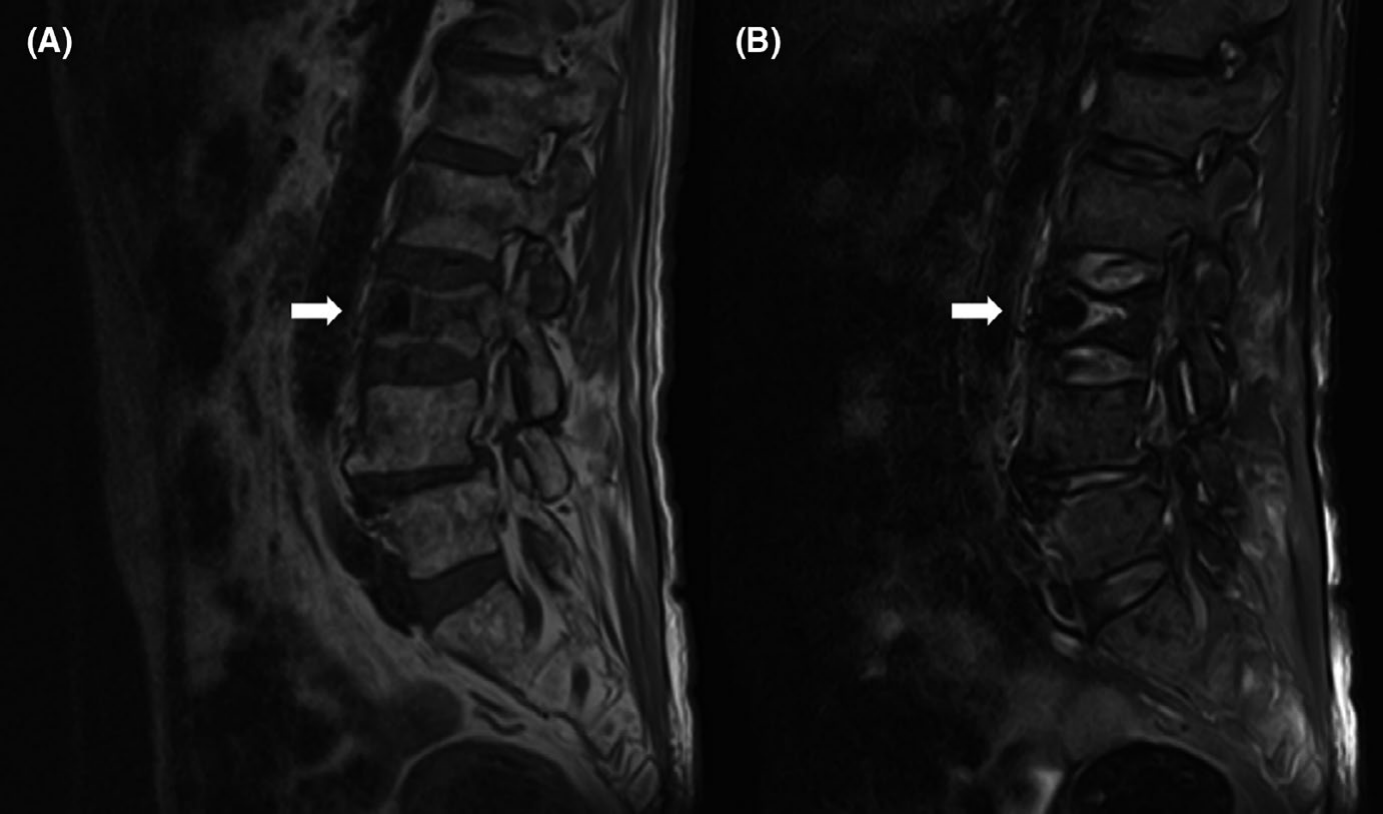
Sagittal magnetic resonance imaging of the lumbar spine, revealing vertebral osteomyelitis of the third lumbar vertebra and a compression fracture, as evidenced by the low‐intensity region on the T1‐weighted image (Panel A, arrow) and the high‐intensity region on the fat‐suppressed T2‐weighted image (Panel B, arrow)
